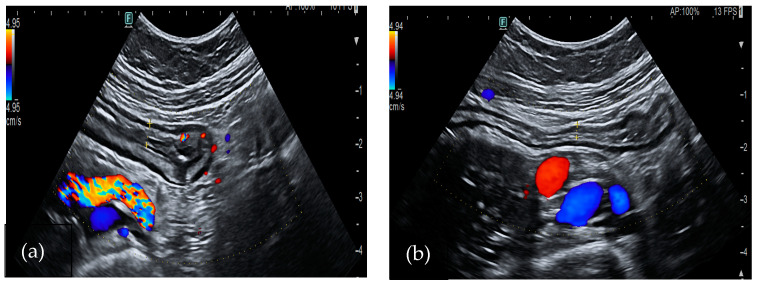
A 36 year-old-woman with Clostridium diarrhea. Bowel ultrasound showed colon wall thickening (a) that normalized after adequate treatment (b).
